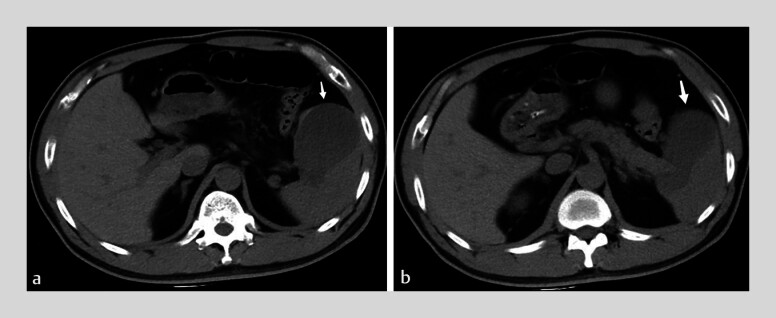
CT demonstrates a pseudocyst at the pancreatic tail adjacent to the spleen (arrows) in a 44-year-old man.
